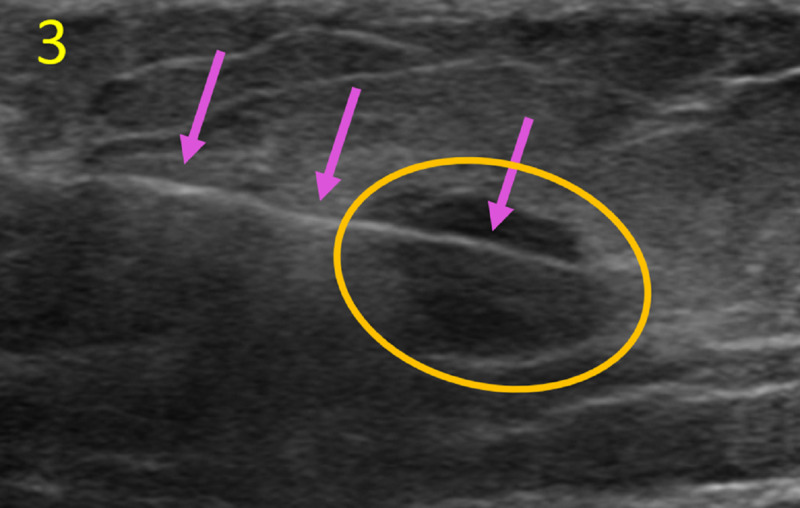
Ultrasound-Guided Biopsy of Right Breast Mass This ultrasound depicts the biopsy needle (pink arrows) sampling the oval right breast mass (orange circle) discovered on screening mammogram. The mass was oriented parallel to the skin with circumscribed margins.
